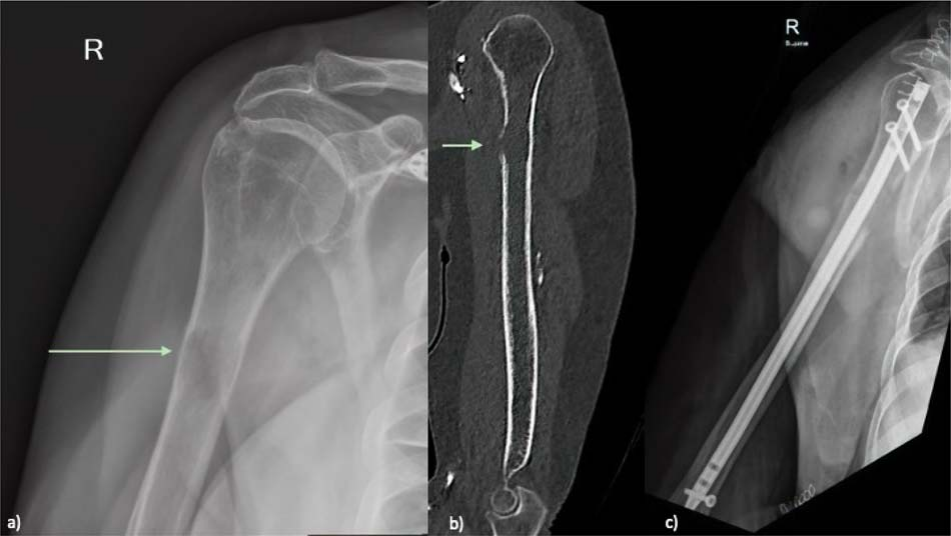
(a) Plain radiograph of the right humerus demonstrating a lucent lesion in the proximal humerus with cortical destruction indicated by green arrow (b) Sagittal CT of the right humerus showing a proximal humeral lesion with breach of the anterior cortex (green arrow) at high risk of pathological fracture (c) plain radiograph Day 1 post prophylactic intramedullary nail of right humerus.
